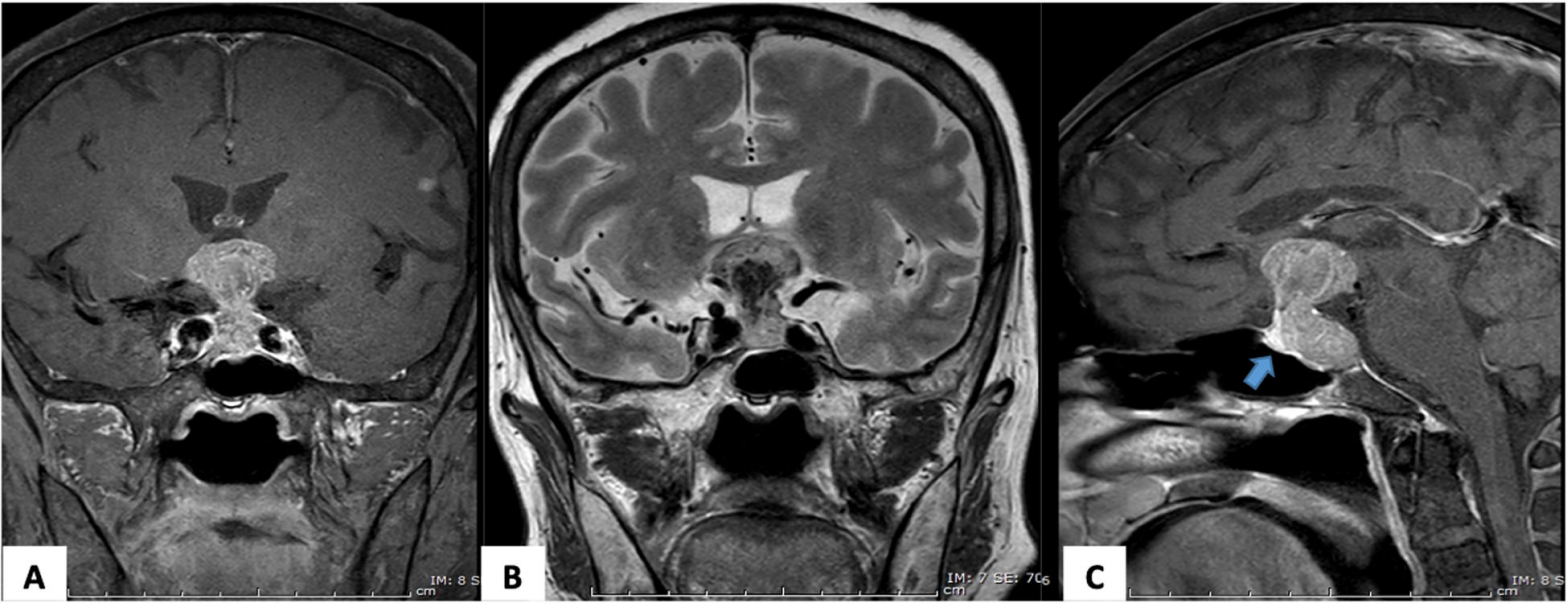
Pituitary MRI with gadolinium contrast. Coronal T1W (a), coronal T2W (b), sagittal T1W (c) images showing a heterogeneous enhancing lesion at sellar and suprasellar regions with a high signal intensity on T1W imaging and a low signal intensity on T2W imaging with central necrosis, abutting the medial aspect of bilateral cavernous sinus. The lesion was compressing the inferior aspect of optic chiasm and anteriorly displacing the residual pituitary gland (arrow)
